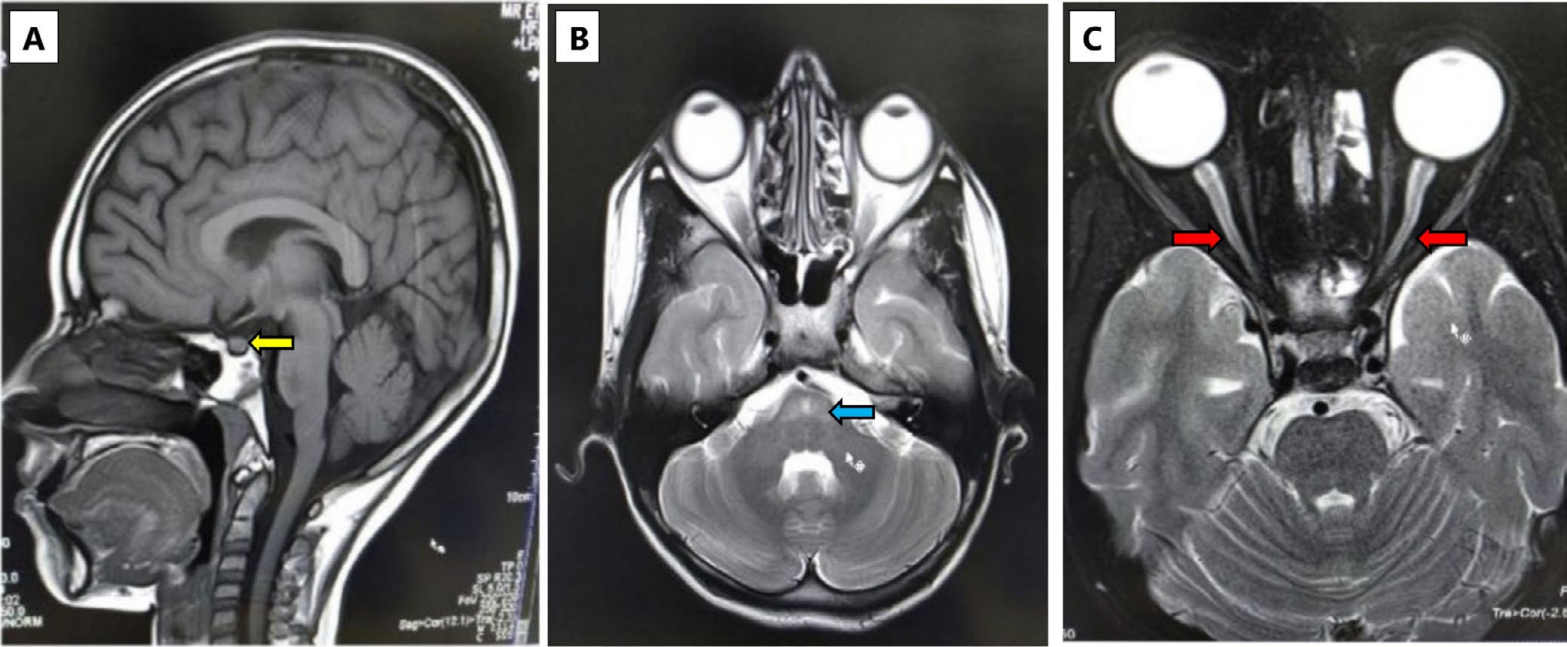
Magnetic resonance imaging brain revealing: (A) (T1 sequence, sagittal view) loss of the normal bright signal (yellow arrow) in the posterior pituitary region; (B) (T2 sequence, transverse view) showing bright, cruciform signals (blue arrow) in the pontine region; and (C) (orbital cuts) showing bilateral optic nerve atrophy (red arrows).
